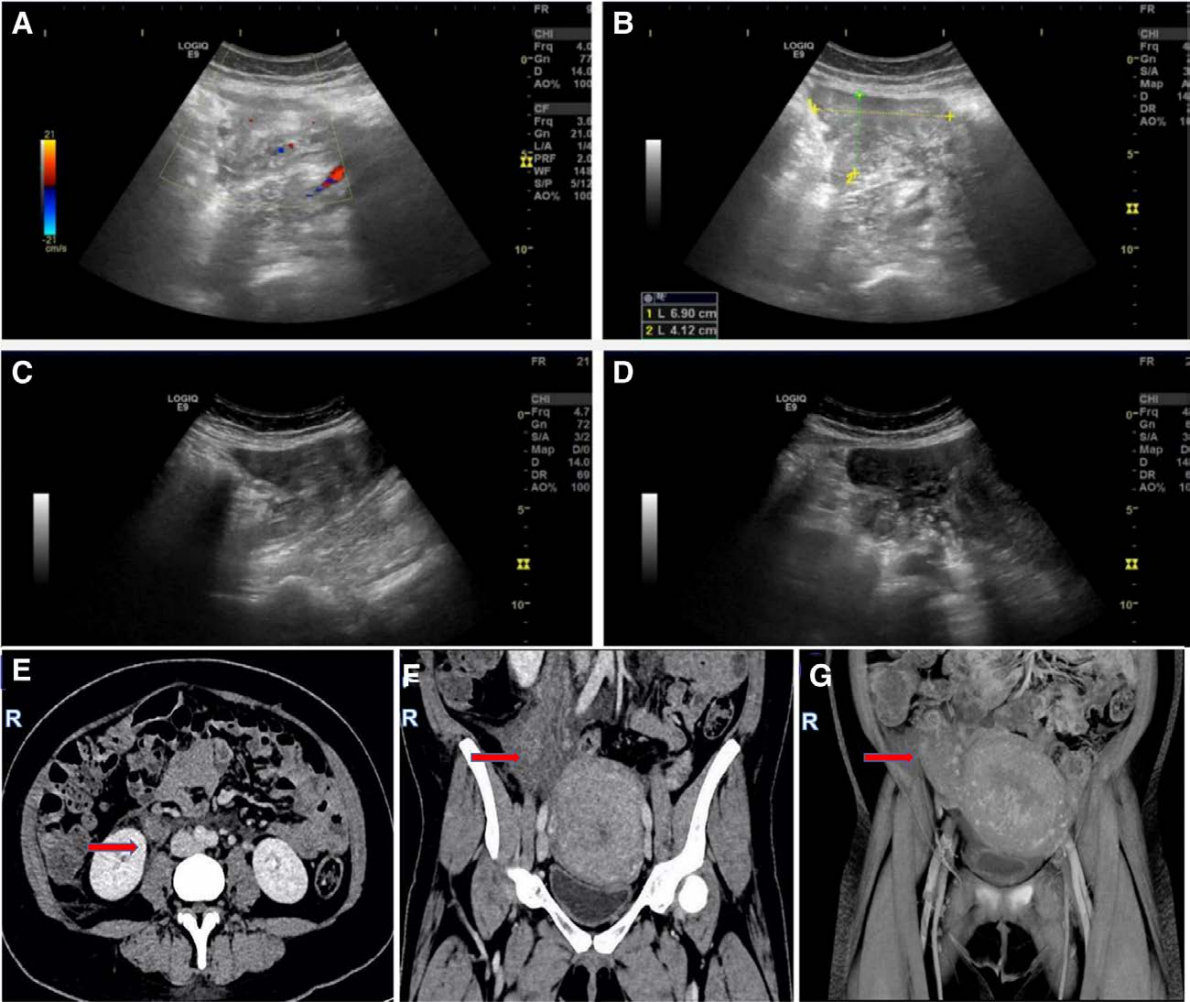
Imaging examination. Day 3 morning US (A and B) 6.9 * 4.1 cm hypoechoic area was detected above the right ovary, with irregular shape, unclear boundary, and a little blood flow signal. Day 3 afternoon US (C and D) 7.2 * 5.3 cm hypoechoic area was detected in the right accessory area, with boundary was unclear and rich blood flow signal, surrounding ovarian. Tubular anechoic structure with thick wall and poor internal sound transmission was detected on the outside. Day 3 CT (E–G) Right ovarian vein thrombosis (The arrow indicates the lesion). US = ultrasound.
